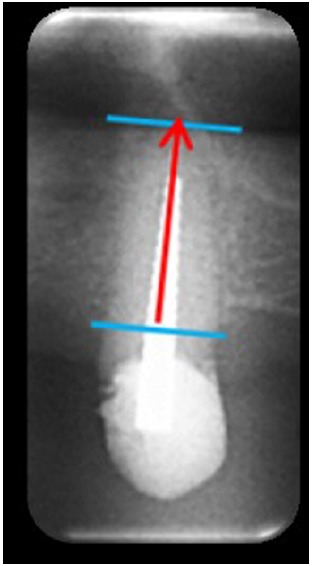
Pre-operative socket height is evaluated as the distance between apex and lowest crestal visible point on a panoramic radiography.
